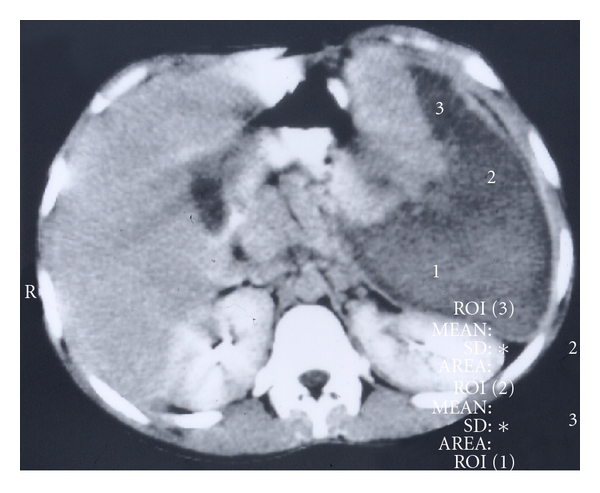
Abdominal CT scan showing massive splenic infarction in a child with sickle cell anemia.
